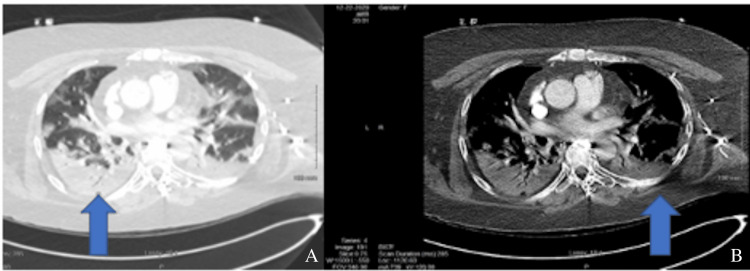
(A) and (B) are thoracic cavity CT scans that show bilateral extensive multilobar airspace disease, which is consistent with COVID-19 pneumonia.
